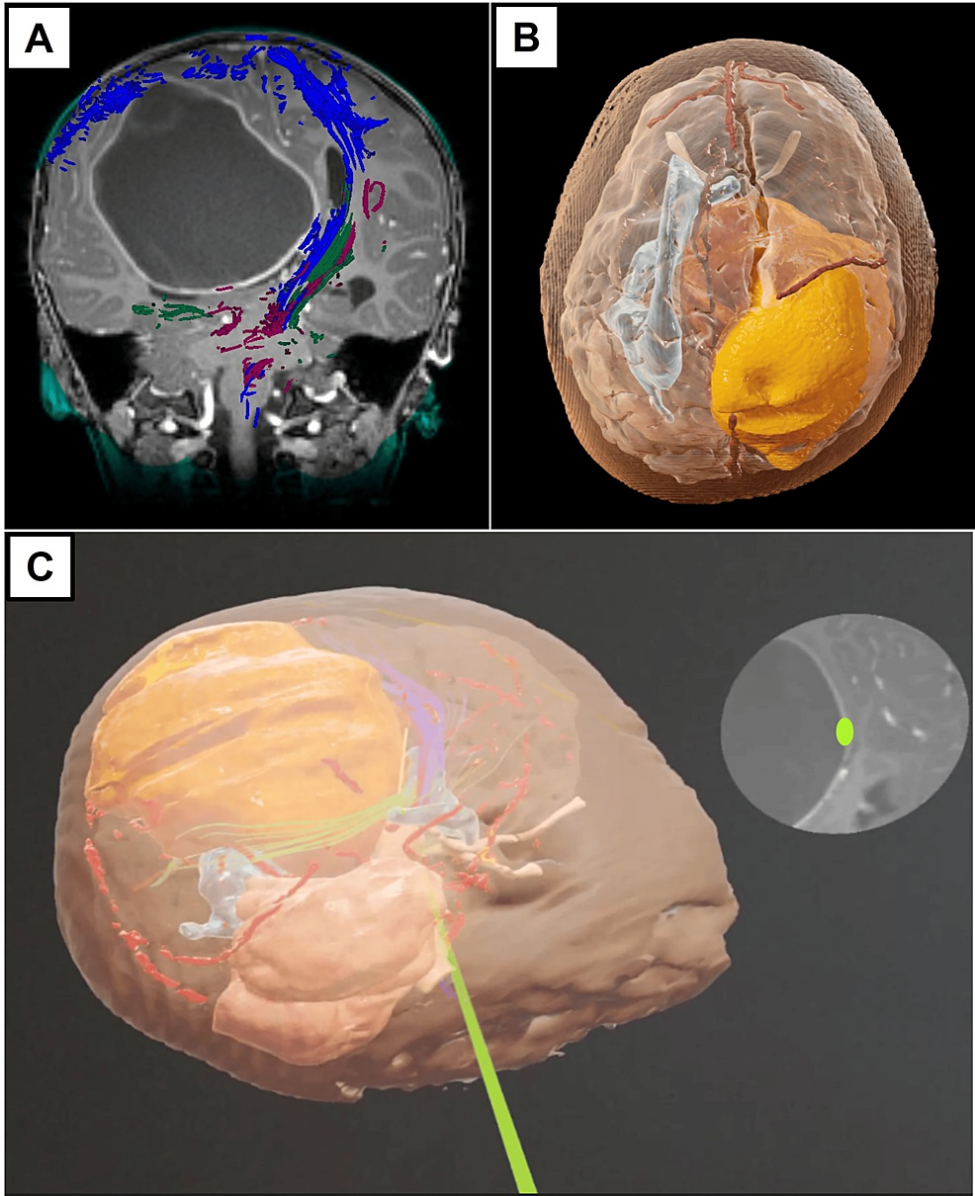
Summary of images used for Case 1. (A) Representative preoperative magnetic resonance imaging (MRI) image in the post-contrast T1-weighted sequence in coronal direction. Here, the right giant supratentorial brain tumor (GSBT) is pushing the adjacent corticospinal (blue), corona radiata (purple), and optic radiation (green) white matter tracts to the left. Of note, tractography is generated via the Modus Plan™ (Synaptive Medical, Toronto, Canada). (B) Representative image of the three-dimensional (3D) model after segmentation of the tumor (yellow-gold) and surrounding normal structures. Here, the tractography is omitted for the purposes of displaying the model’s anatomical details. (C) Representative streamed augmented reality (AR) images placed next to the corresponding MRI brain slide to allow the neurosurgical team to know the location of the brain-tumor interface posterior to the adjacent corticospinal tract (light green).
